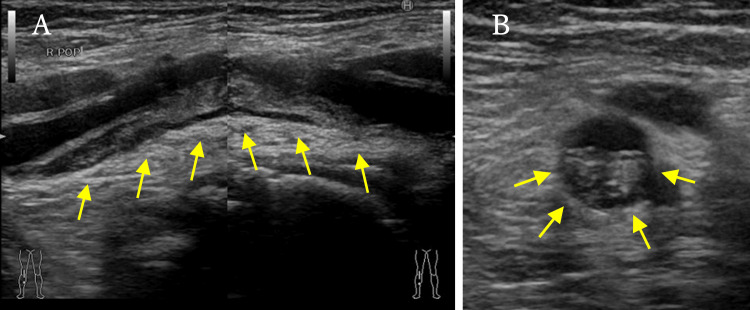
Ultrasonography of arteries in the lower extremity. (A) An iso-echoic lesion is located in the right popliteal fossa (arrows). (B) The lesion narrowed the lumen of the blood vessel (arrows).
